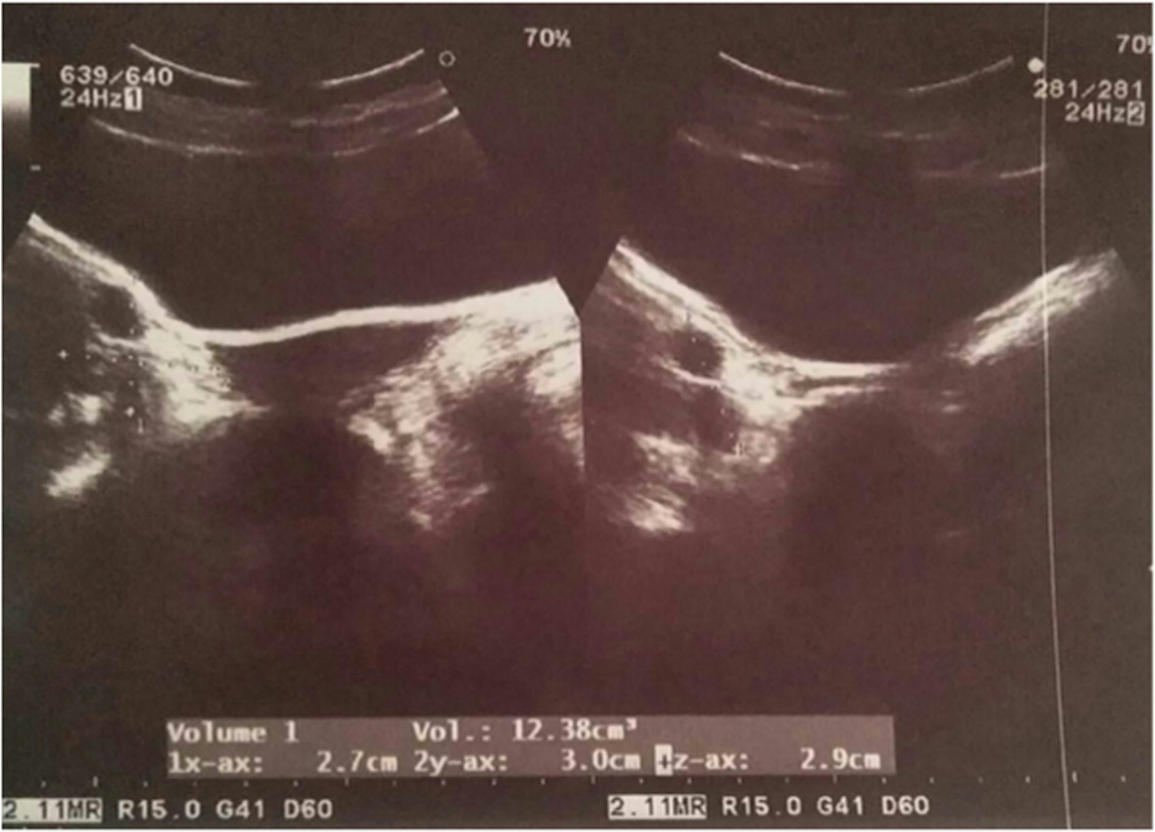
Trans‐abdominal ultrasound shows an enlarged right ovary with multiple cysts
